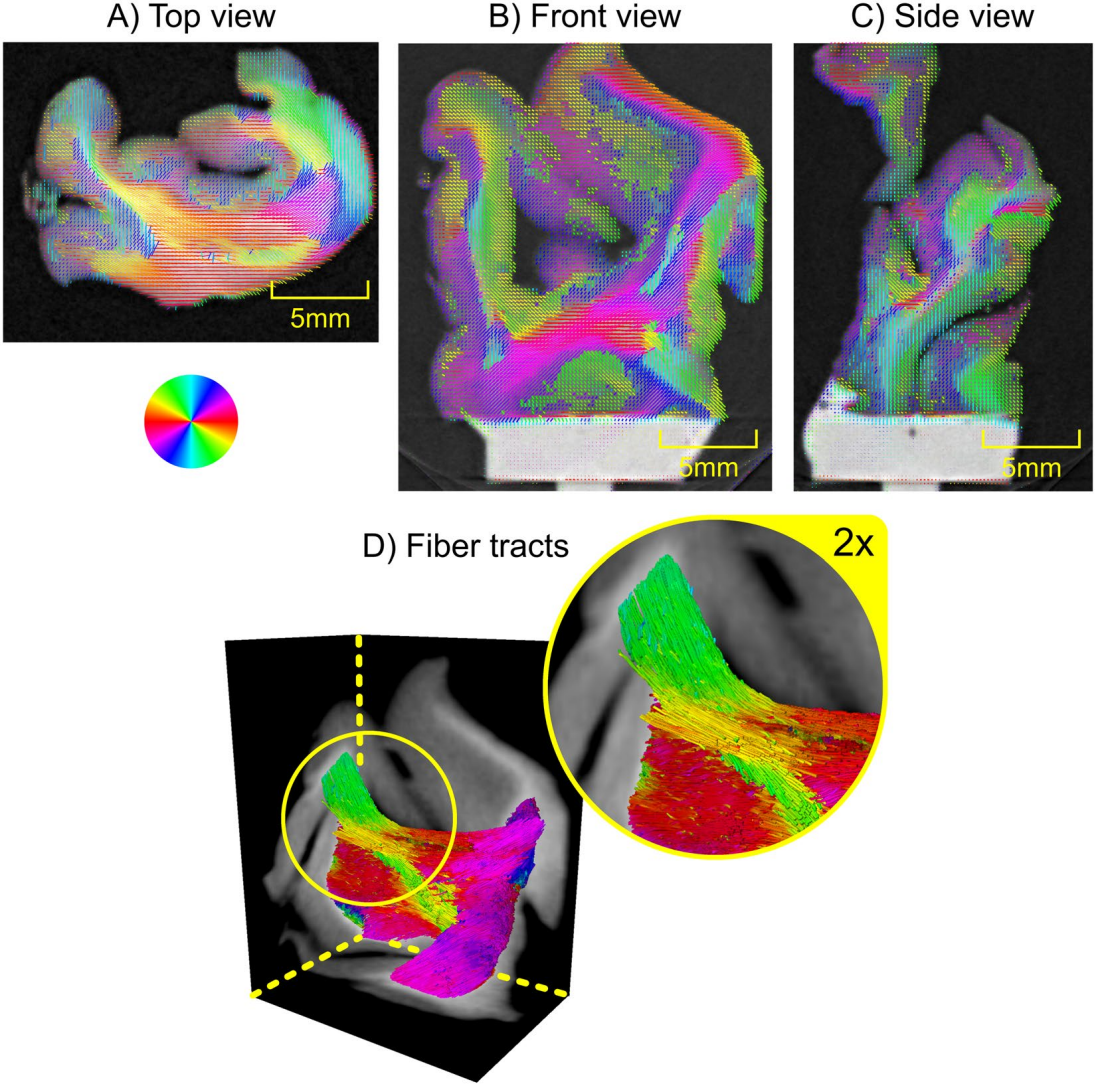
(A–C) Three slices of the attenuation X-ray CT overlaid with the fiber tract orientations as produced by our approach of Anisotropic X-ray Dark-field Tomography (AXDT). The coloring shows the in-plane orientation according to the color-wheel at the top left. In (D) we display three cross-sections of the 3D attenuation X-ray CT overlaid with the fiber tracts within the white matter as computed with a streamline method. The coloring was chosen based on the orientation of the tracts projected onto the plane orthogonally to the viewing direction according to the color-wheel.
